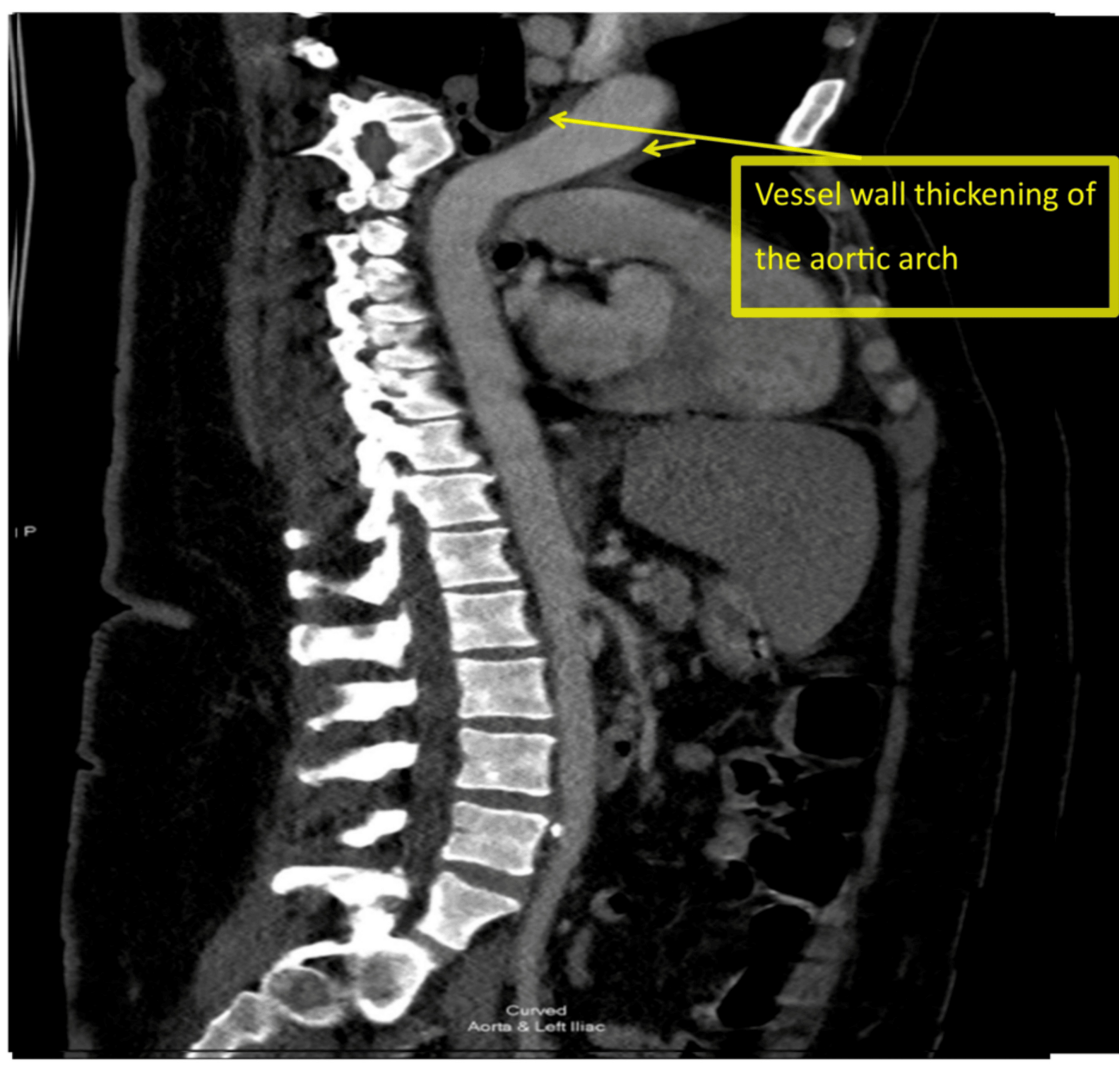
Sagittal section of CT aortography showing vessel wall thickening of the aortic arch
